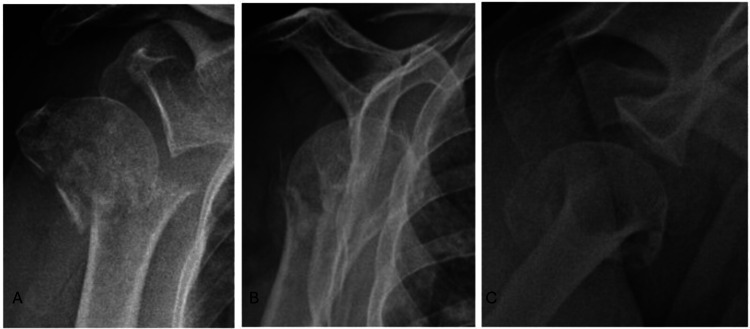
Preoperative radiographic images of the patient in the following views: true anteroposterior (A), scapular profile (B), and Velpau axillary (C) observing the fracture lines in the proximal humerus
